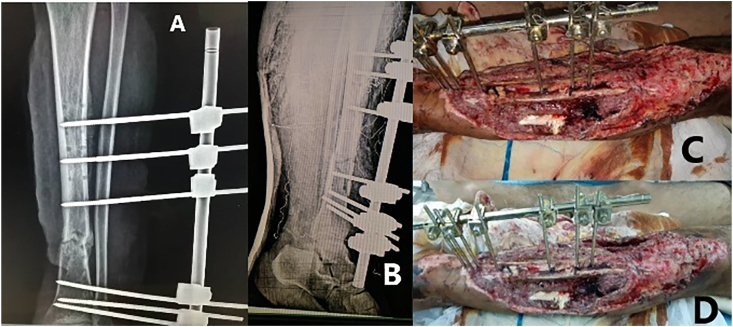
After surgery; anterior (A) and lateral (B) tibial X-ray show external fixator type AO with pins. postoperative clinical photographs showing (C, D) open tibial fracture with soft tissue defect and wasting of the anterior compartment muscles.
